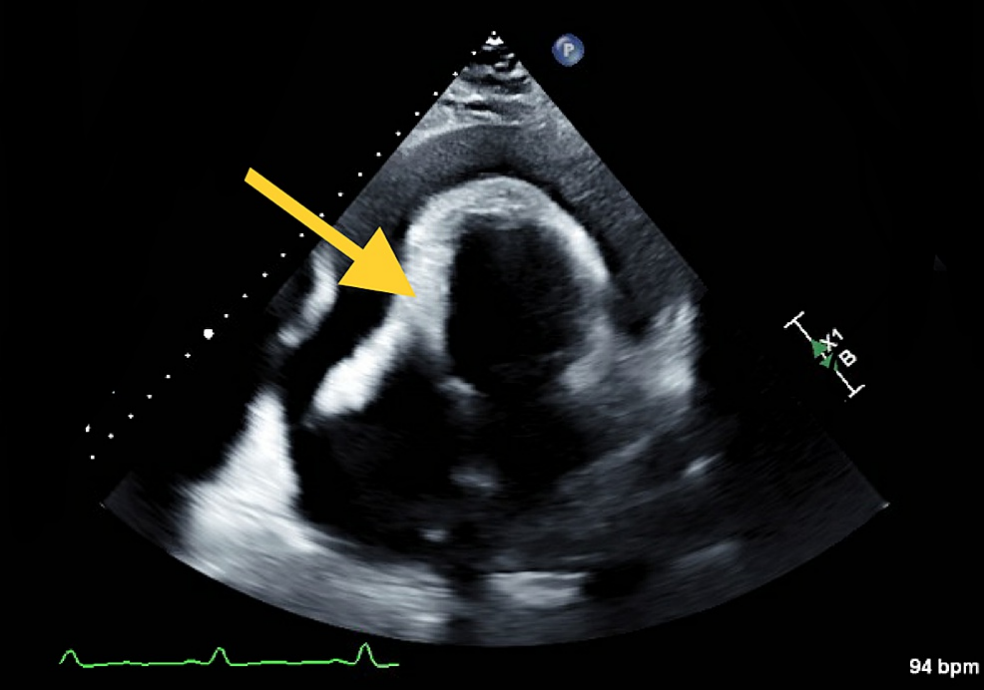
Transthoracic echocardiogram with noticeable large effusion and the yellow arrow showing the right ventricular collapse
